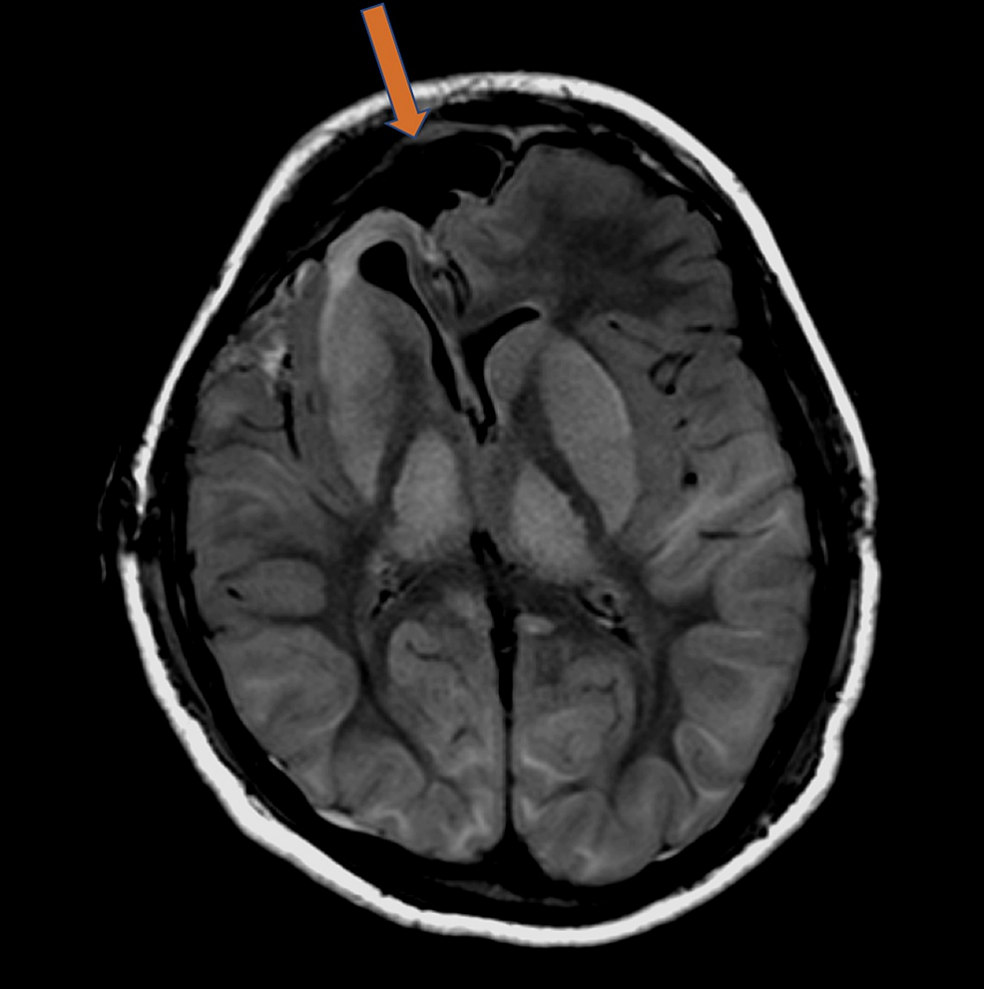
FLAIR image, postoperative day 0
